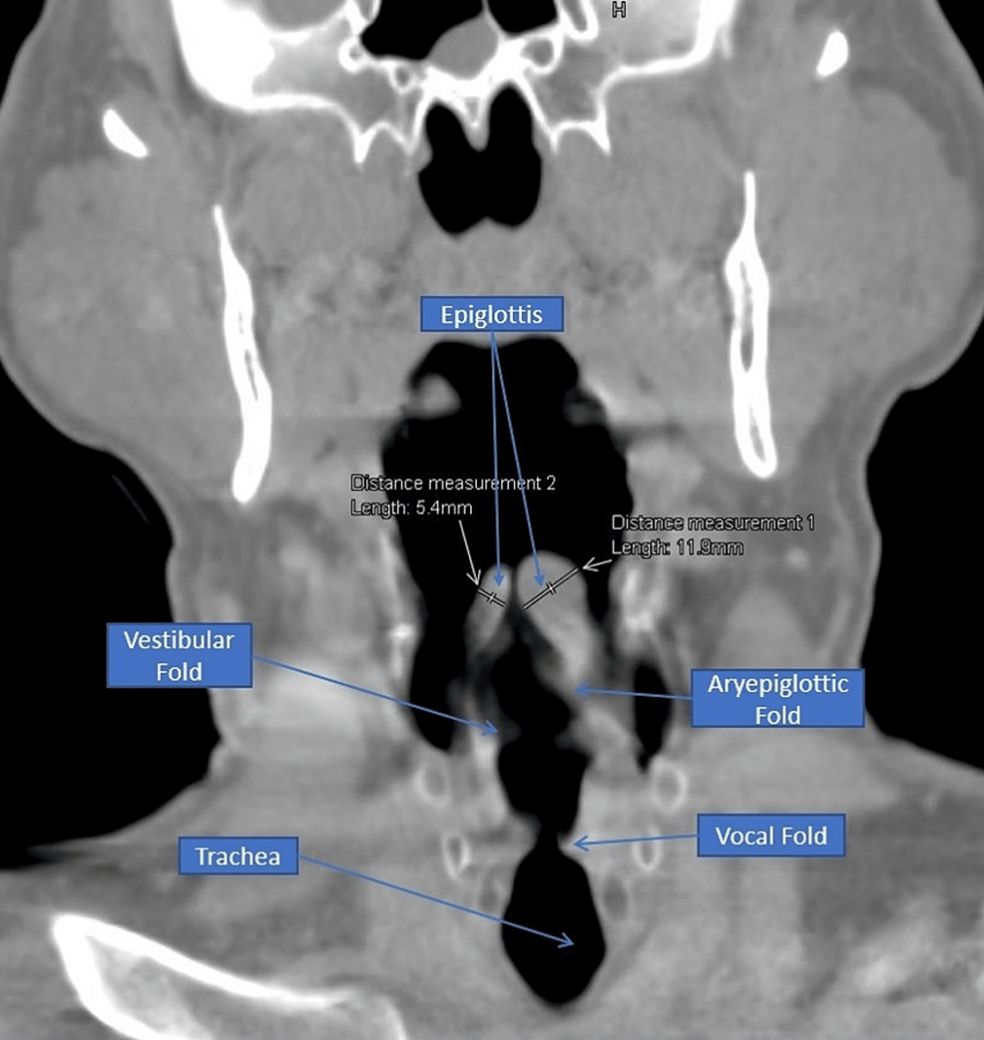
Coronal section of the CT scan neck with contrast CT: Computed Tomography
